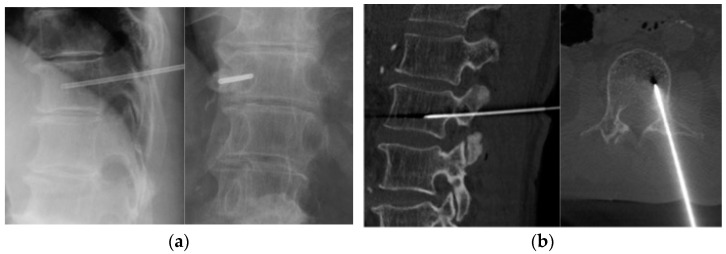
Image-guided percutaneous needle biopsy. (a) Fluoroscopic biopsy. (b) CT-guided biopsy.
